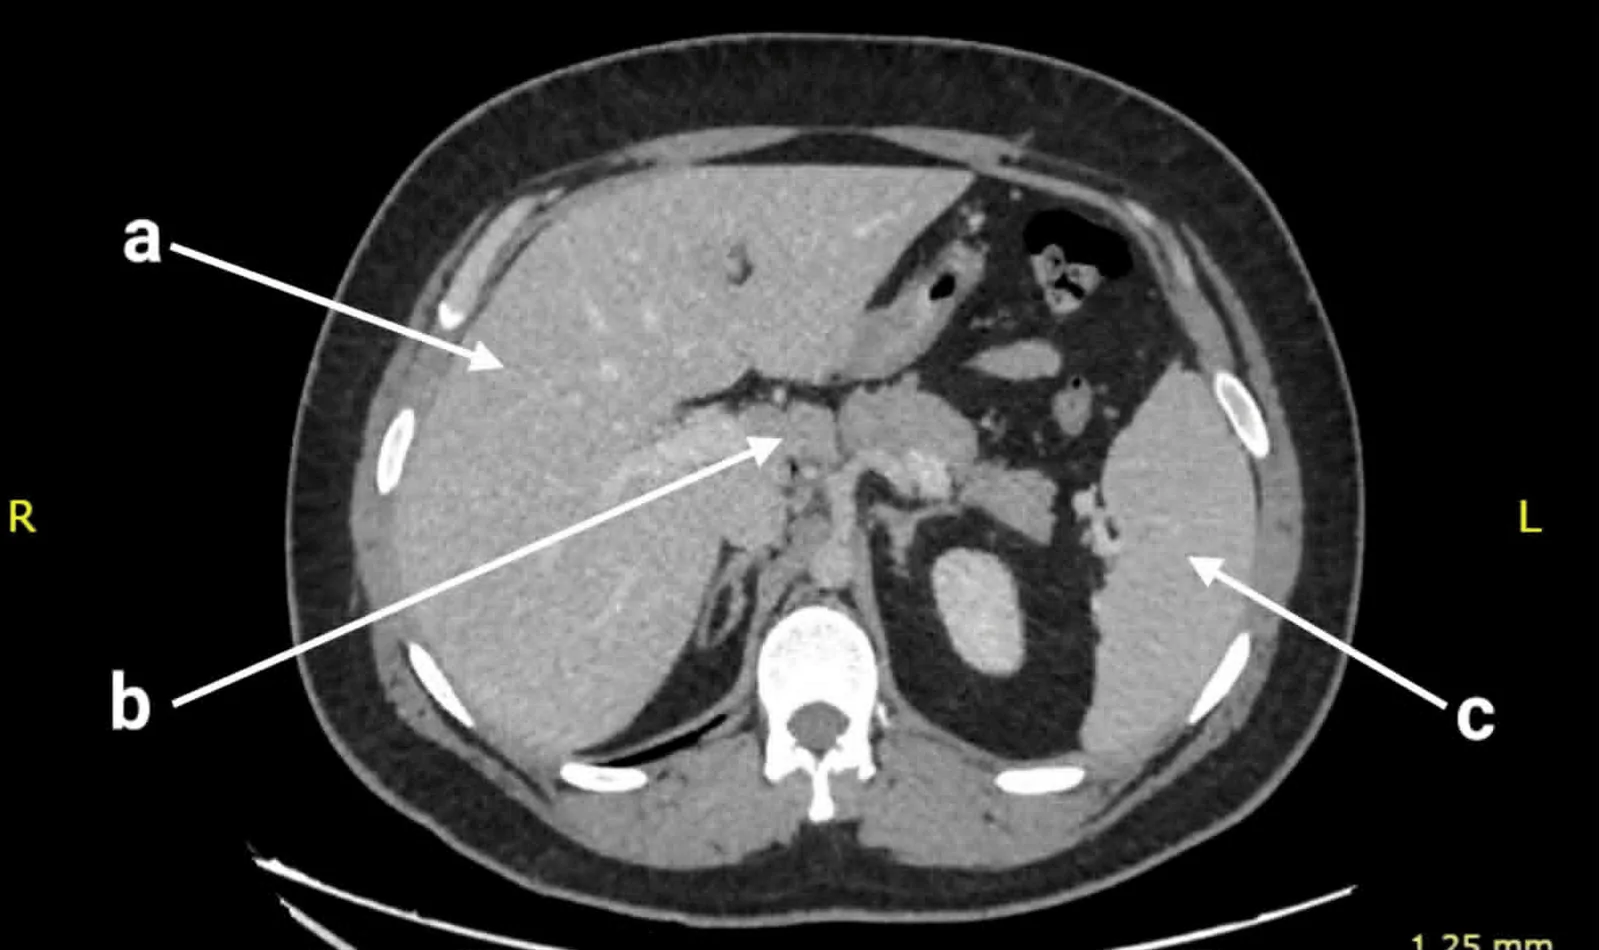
Contrast-enhanced computed tomography of the upper abdomen Axial contrast-enhanced computed tomography demonstrates (a) hepatomegaly with mildly heterogeneous hepatic enhancement, (b) enlarged lymph nodes at the porta hepatis, and (c) splenomegaly. The intrahepatic bile ducts are not dilated.
